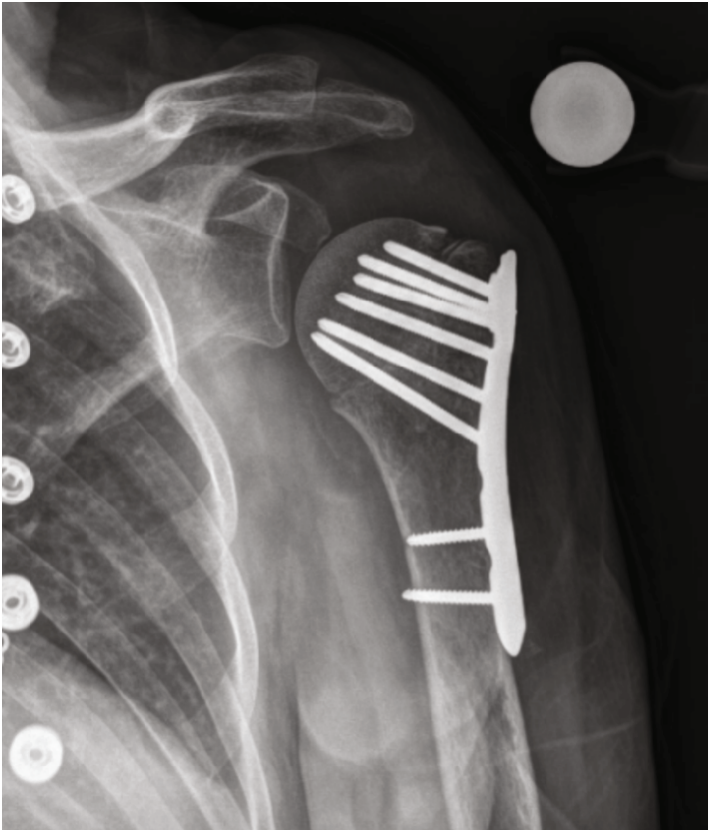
Left proximal humerus fracture following open reduction and internal fixation. A plain radiograph of the left shoulder taken in the anteroposterior (AP) direction six weeks after surgery. There is no displacement of the fracture fragments and reduction is satisfactory.
